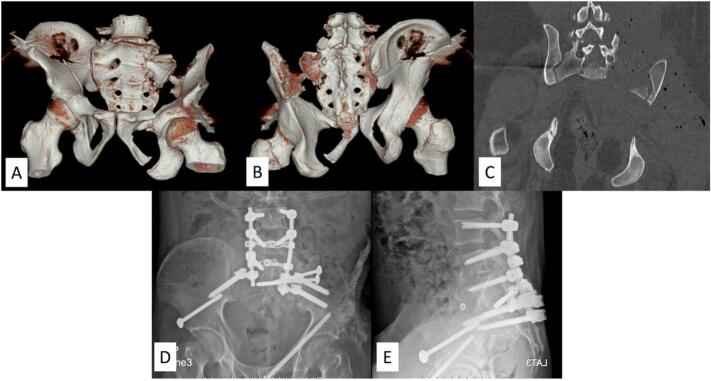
Demonstrating an AO type C2 sacral fracture, Isler subtype 2b (case No. 2). (A-C) Preoperative CT scan and CT reconstruction images showing left L5S1 facet joint subluxation and comminution of the right L5S1 facet joint. In addition, this patient had an open pelvic fracture with a following infection that required multiple debridements, resulting in severe bone loss of the left iliac wing. (D-E) Postoperative radiographs after lumbopelvic fixation to achieve secure fixation.
